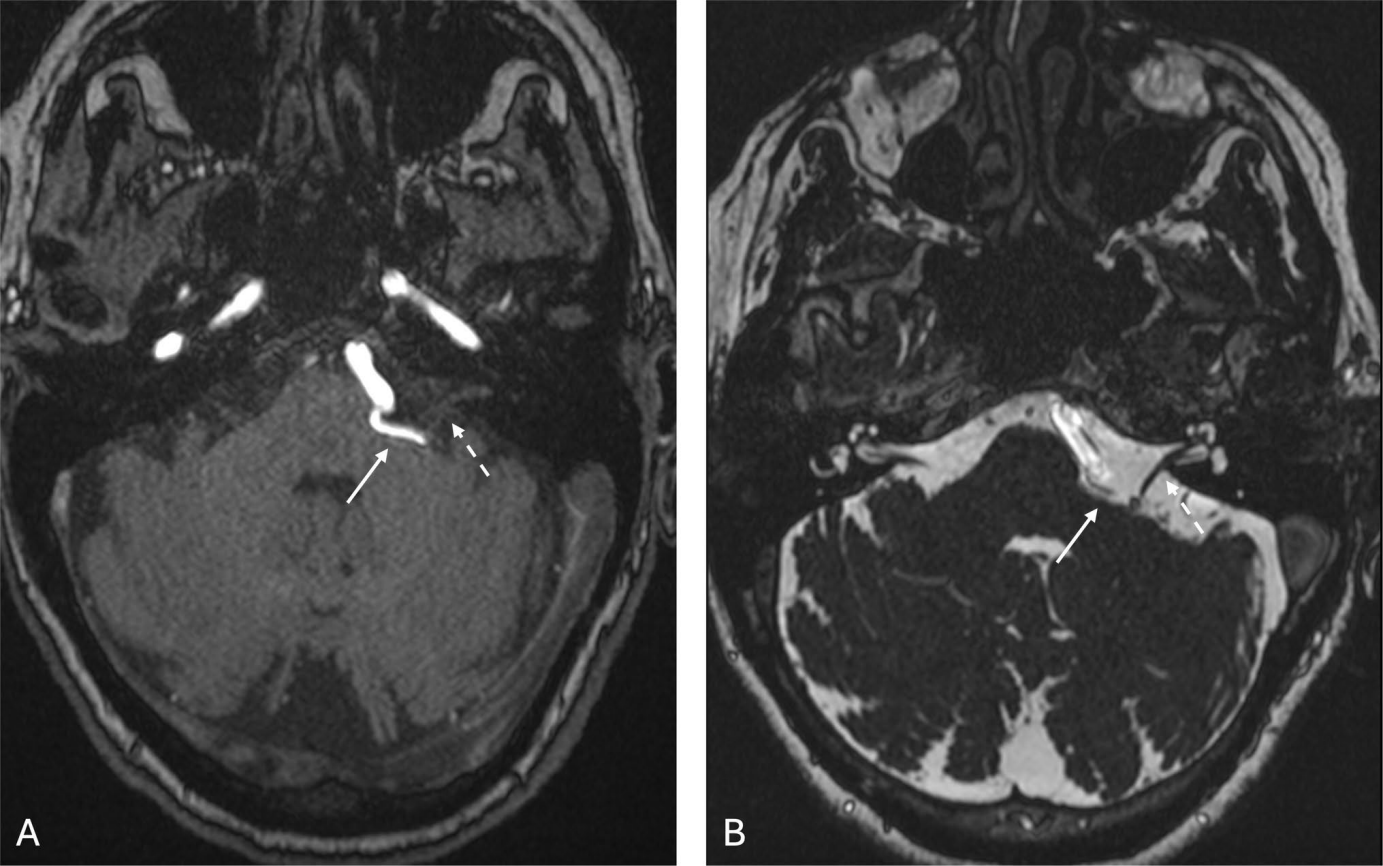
Preoperative MRI of the brain: TOF (A) and CISS (B) sequences. A segment of the Anterior inferior cerebellar artery (white arrow) compresses the facial nerve (white dotted arrow) in the left cerebellopontine angle
